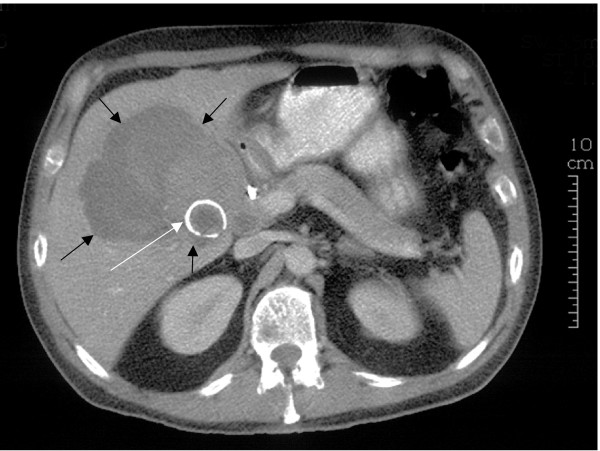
Abdominal computed tomography scan. White arrow, ring calcified lesion, gallstone. Black arrows, extent of haematoma.
